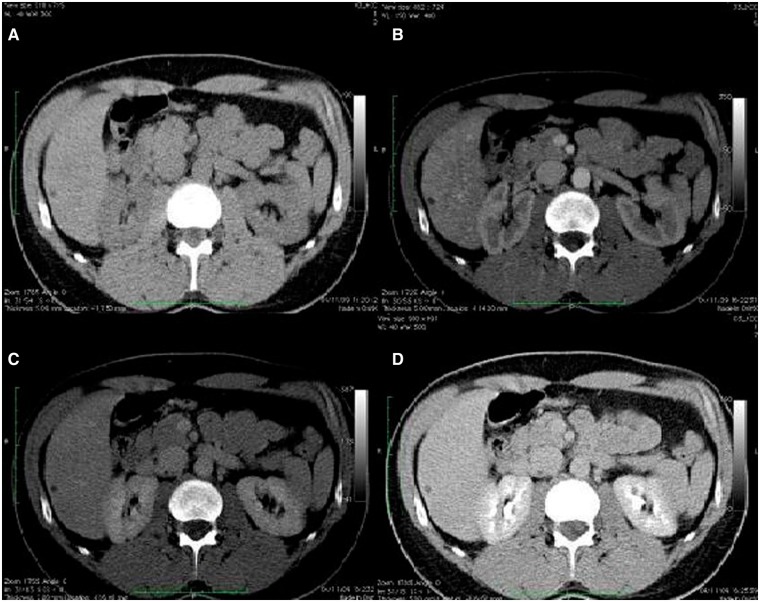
Triphasic computed tomography scan showing no focal liver lesion. Basal (A), arterial (B), portal (C) and venous phase (D) showing no focal liver lesion or no sign of diffuse liver disease except for a cyst at the left lobe of the liver.
